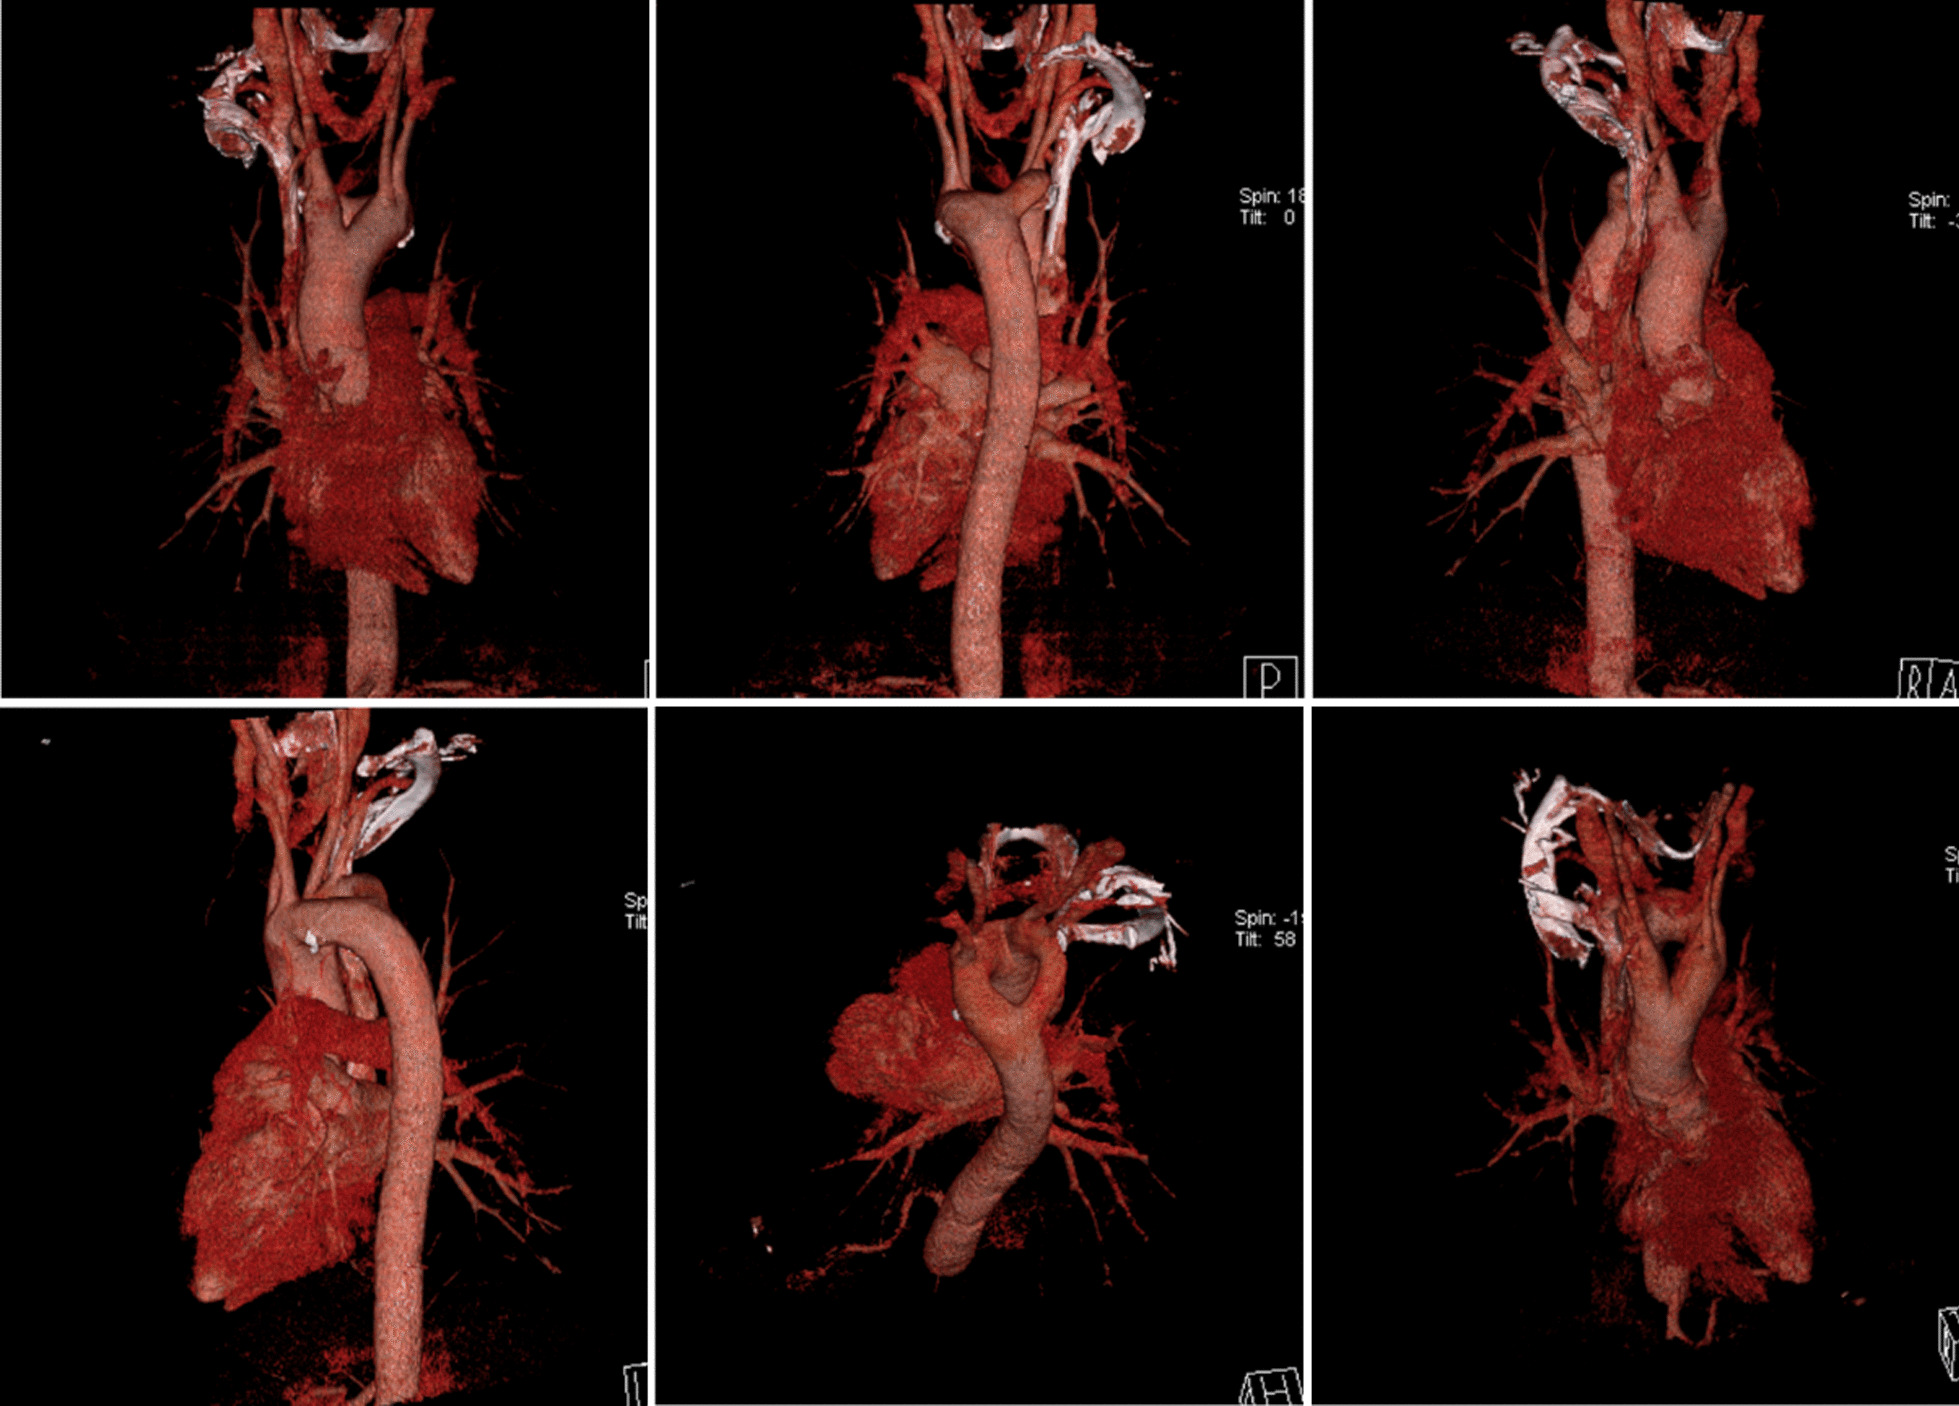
Three-dimensional computed tomography (3D-CT) Scans Show a DAA in different directions
